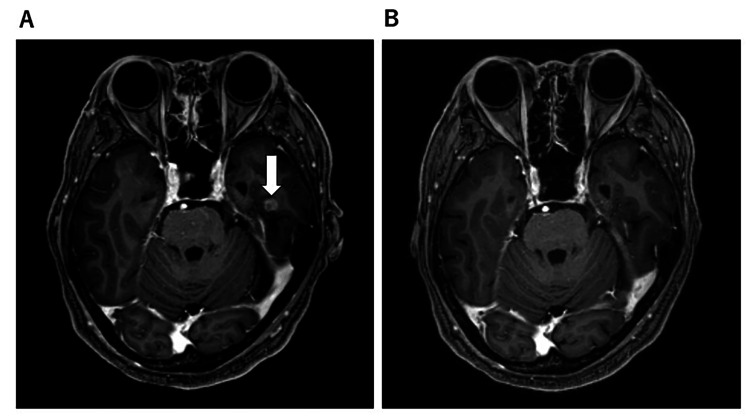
Brain metastases on brain MRI A: - 13 days. Metastatic lesion 1 cm in diameter in the medial part of the right temporal lobe. B: 88 days. Metastases have disappeared. The white arrow indicates cancer.
